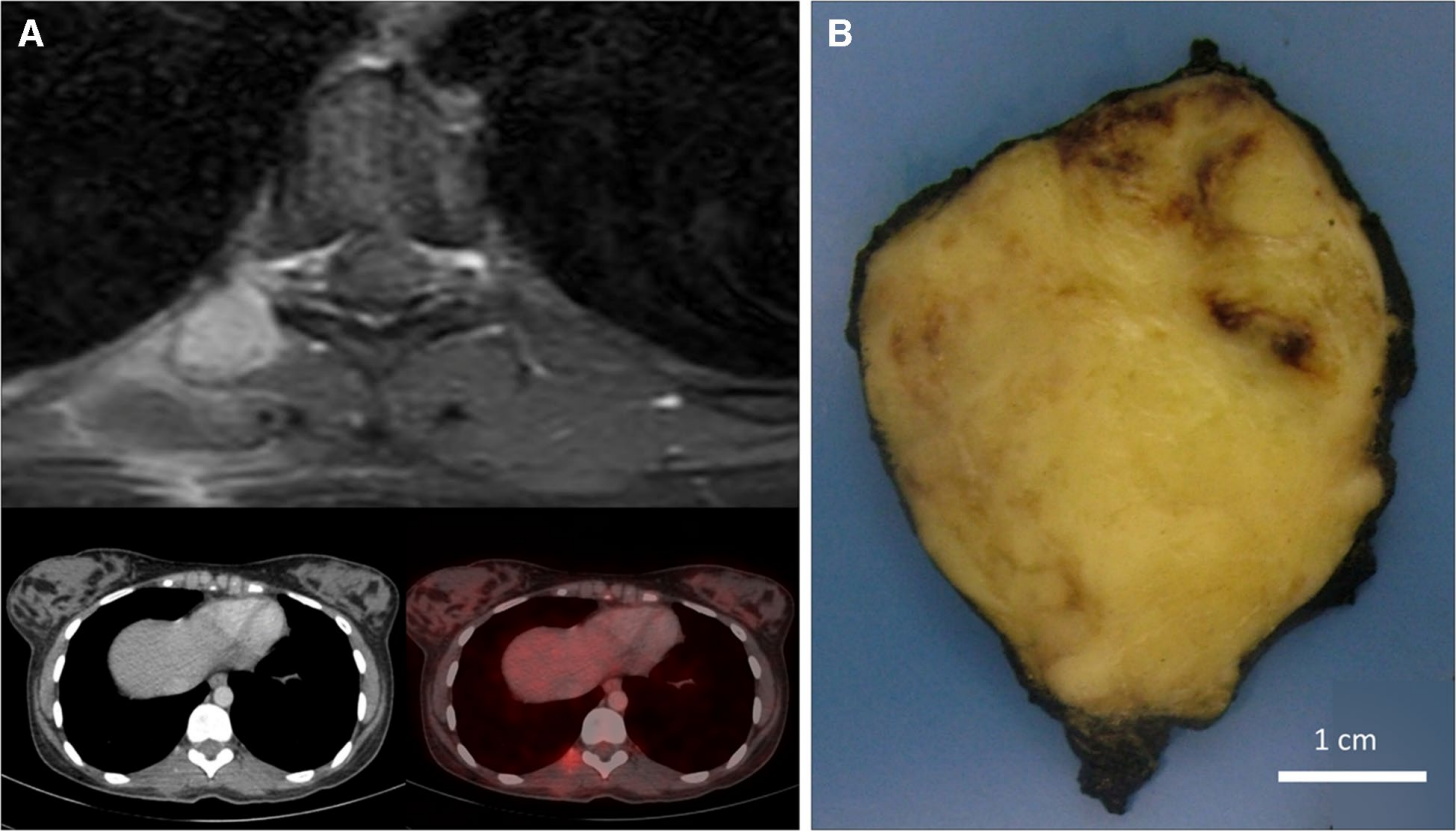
A Case 1. Magnetic resonance imaging (MRI) showing a 7.5-cm diameter soft tissue tumor in the right paraspinal region and corresponding positron emission tomography-computed tomography (PET-CT) images. B Case 2. Marginal excision of a 6-cm diameter soft tissue tumor with homogeneous myxoid cut-surface with foci of hemorrhage. Note the presence of small nodular extensions in pseudocapsule
